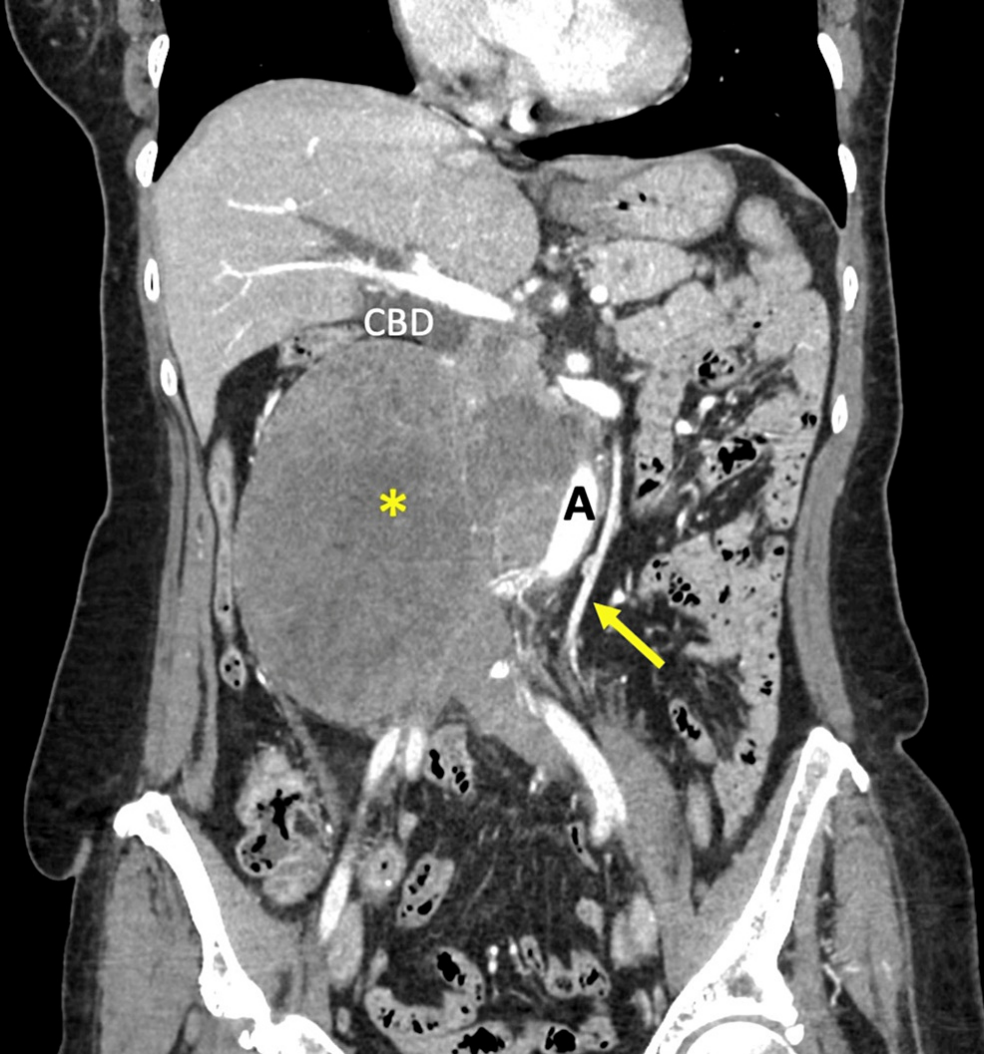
Coronal CT images The large mass (asterix) displaces the abdominal aorta (A) and superior mesenteric vessels (arrow) to the left. Dilatation of the common bile duct (CBD) is visible.
